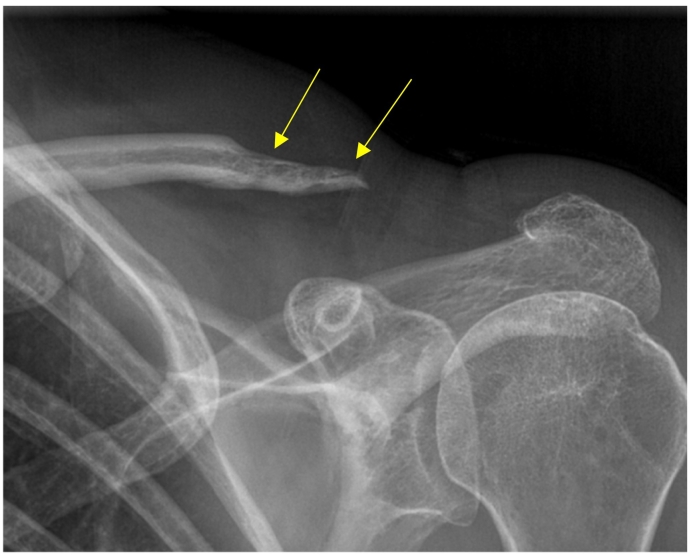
One month anteroposterior follow-up radiograph demonstrating continued osteolysis (yellow arrows) despite resolution of symptoms and no remaining limitations in function status-post revision surgery. (For interpretation of the references to colour in this figure legend, the reader is referred to the web version of this article.)
